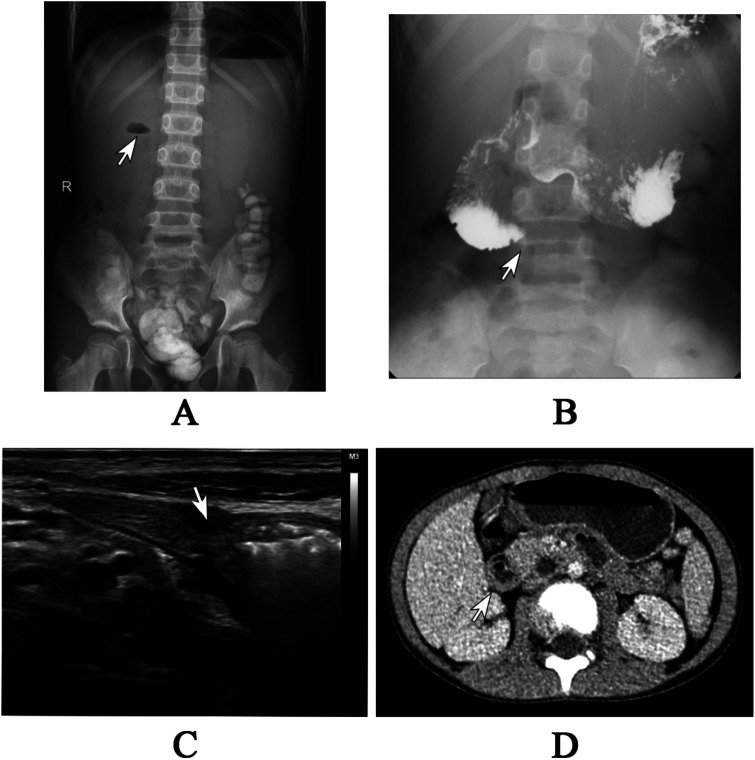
(A) The plain x-ray revealed a small air-fluid level in the upper right quadrant, accompanied by the absence of gas in the distal area. (B) The barium meal study demonstrated an obstructed passage of barium in the third segment of the duodenum. (C) The abdominal ultrasound showed a strip-like hypoechoic external compression affecting the third part of the duodenum, preventing the passage of contents. (D) The abdominal CT scan showed the distension of proximal duodenum with fluid accumulation.
